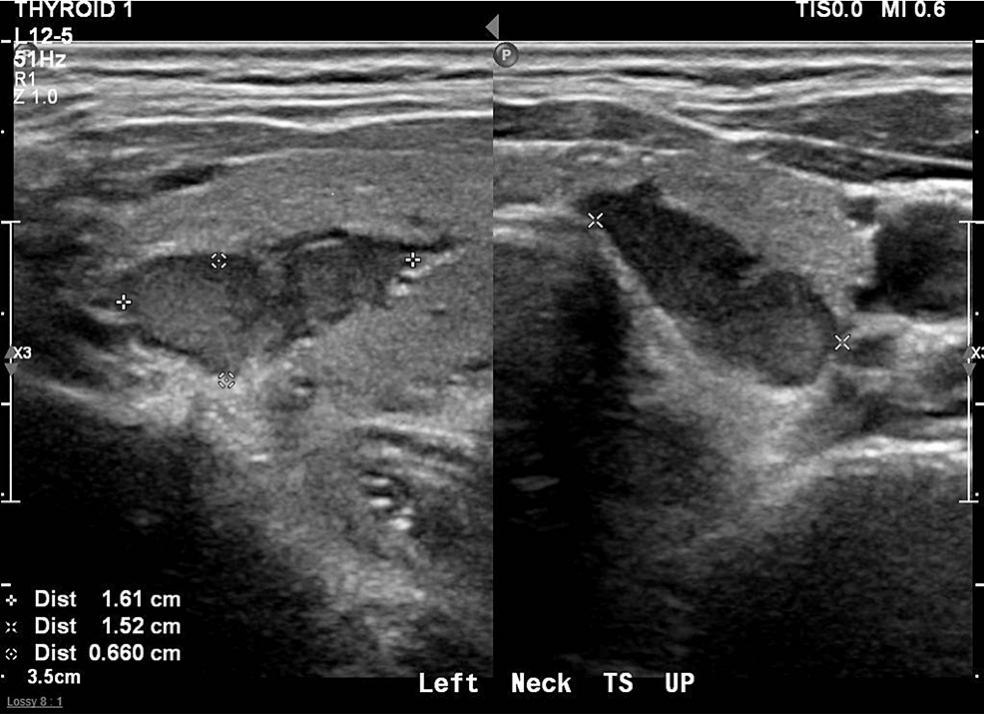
Ultrasound using a linear probe demonstrated a hypoechoic, well-defined, solid lesion at the left upper neck adjacent to the left lobe of the thyroid.
